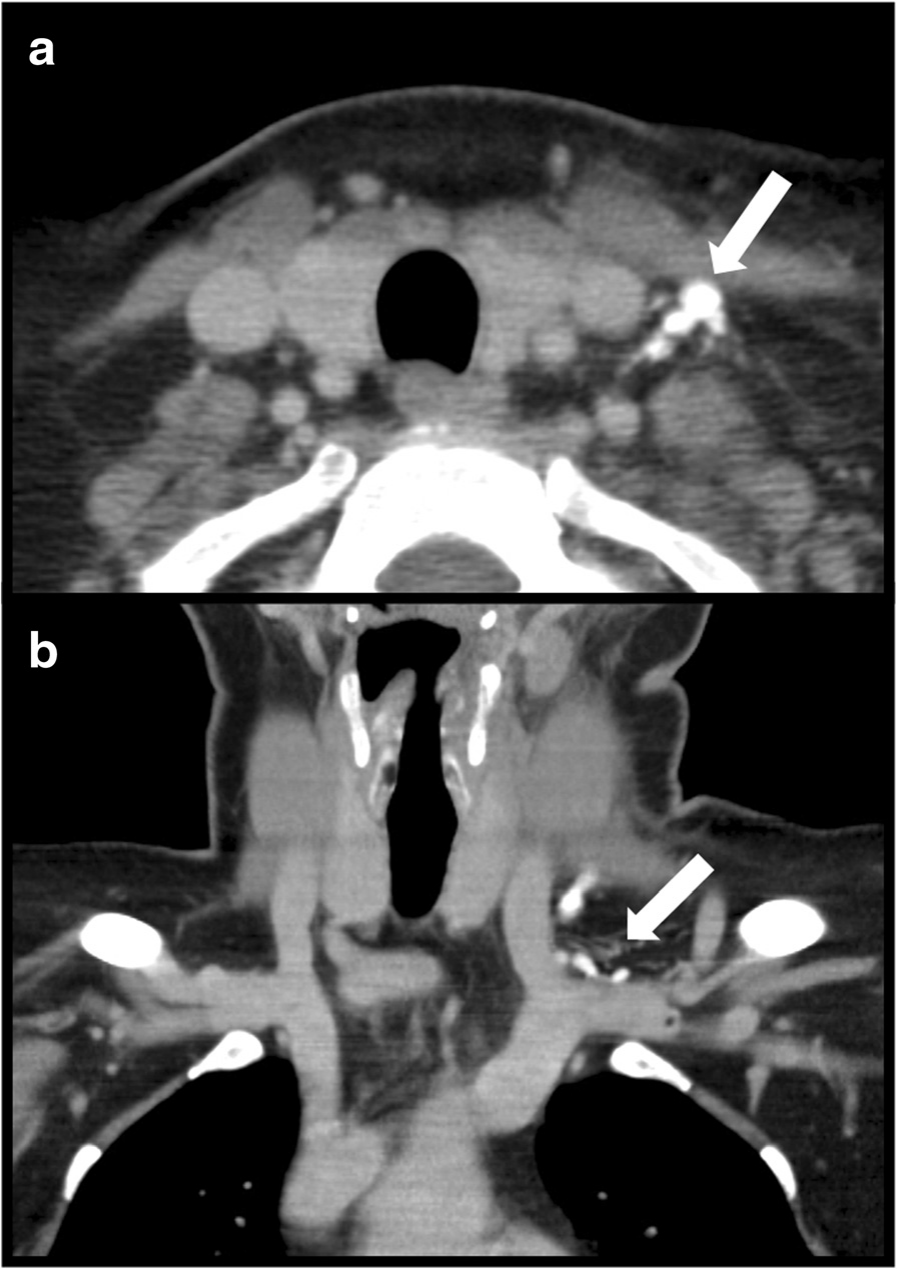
A 57 year old female with parotitis. Contrast enhanced computed tomography in the axial (a) and coronal (b) plane. Reflux of contrast media into a dendritic configured thoracic duct (arrows)
